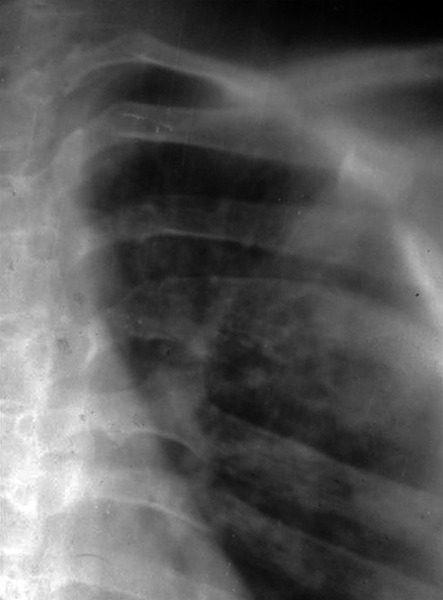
Chest radiograph showing unilateral bifid 5th rib
